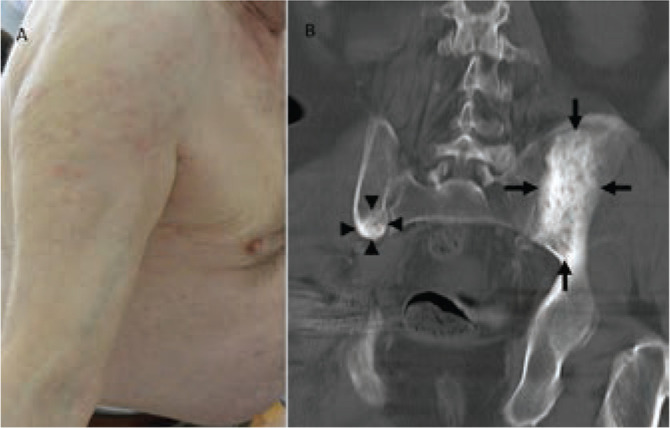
(a) Skin rash with urticaria-like features. (b) Reformatted coronal oblique CT image (bone windows) at the level of the sacroiliac joints. Centred at the left ilium, there is a predominantly sclerotic lesion with lace-like pattern, narrow zone of transition and relatively well-defined margins (arrows). Note the presence of a similar smaller lesion on the right iliac bone (arrowheads).
